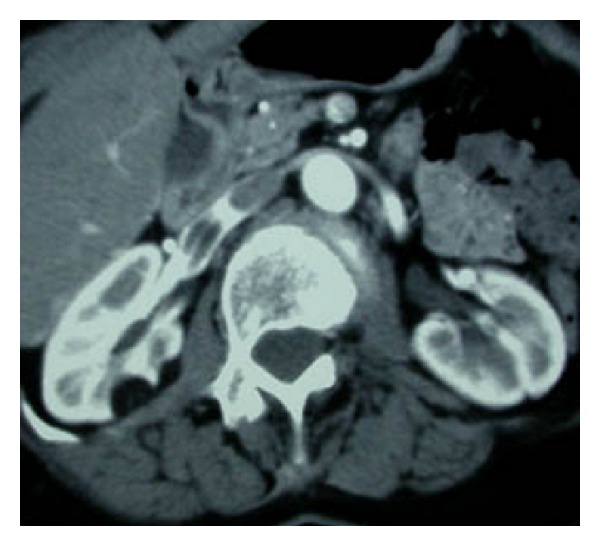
Axial contrast enhanced abdominal CT showing an IVC and renal thrombus with attenuation value—70 HU.
